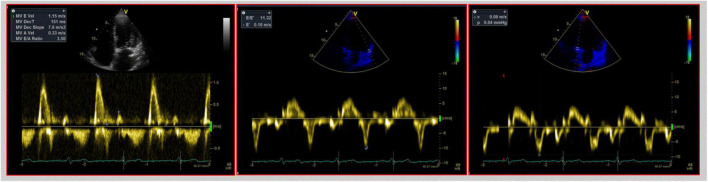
Echocardiographic assessment of diastolic function in heart transplant. This figure shows a mitral E/A ratio ≥2 with a deceleration time of E wave of 151 ms, thus indicating a possible restrictive filling pattern in a 3-year heart transplant patient. However, TDI analysis, shown in the middle and right pictures, shows normal e’ lateral and septal velocities, thus possibly excluding a restrictive filling pattern.
